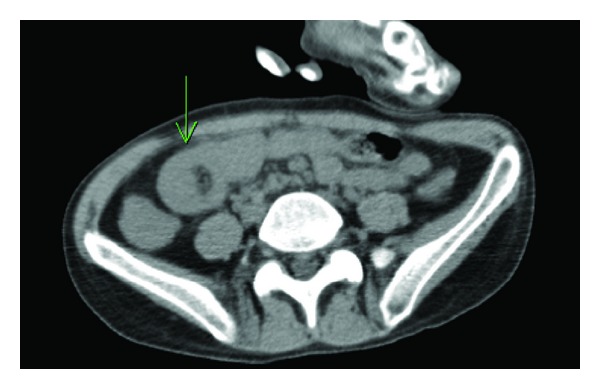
Computed tomography of the abdomen and pelvis revealing concentric, alternating layers of hyperechoic and hypoechoic shadowing within the intestine, suggestive of a duodenojejunal intussusception (arrow).
